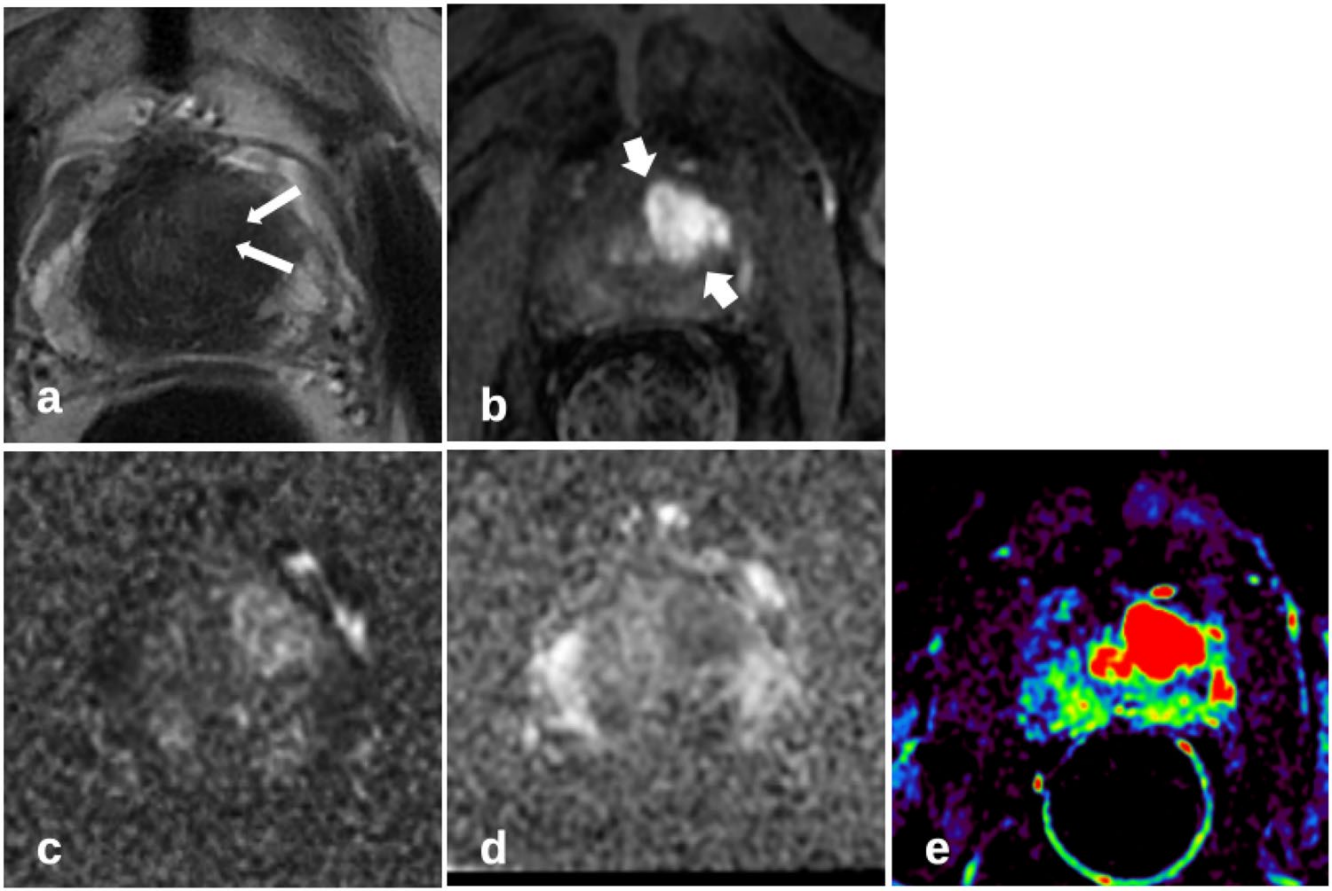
Typical tumor perfusion pattern (short arrows in b) of the left anterior transition zone, with marked asymmetry and with marked high signal intensity, moderate reduced heterogeneity; patient of 70 years, PSA 39 ng/ml, prostate volume 36 ml, prostate carcinoma G2 Gleason 3+4; a T2-weighted axial imaging with marked biopsy traces (long arrows); b T1-weighted early-phase dynamic perfusion; c diffusion-weighted imaging with b = 1400; d apparent diffusion coefficient map; e: calculated dynamic enhanced integral EI colour map
